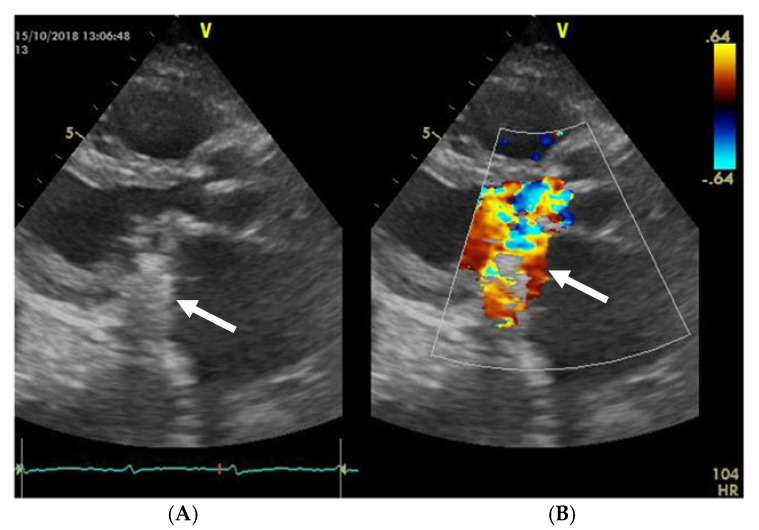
(A) TTE. Prosthetic mitral valve PLAX thrombus on the disc left atrial side (arrow). (B) TTE. Colour Doppler turbulent flow above the prosthesis (arrow).
